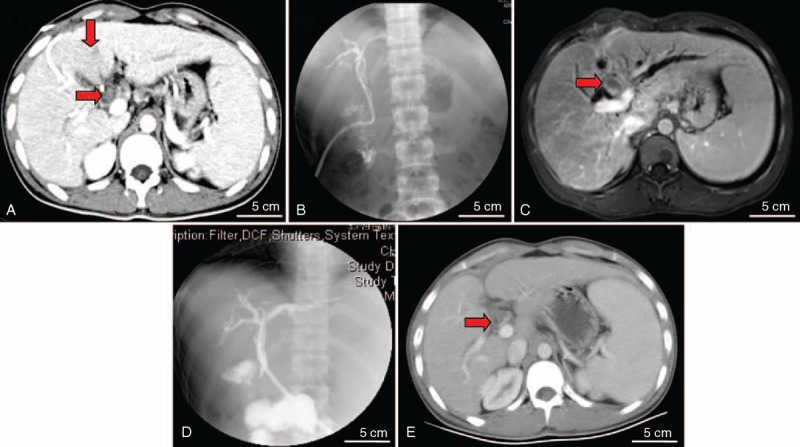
Image data of the patient who experienced BDTT re-recurrence after surgery. (A) HCC at segment 4 (vertical arrow) and tumor thrombus in common hepatic duct (horizontal arrow) were found with computed tomography. (B) Cholangiography showed that no definite filling defect was seen in the biliary system after hepatectomy and thrombectomy. (C) BDTT recurred (horizontal arrow) 7 months after first surgery (observed with functional magnetic resonance imaging). (D) Cholangiography showed that the recurrent BDTT was removed completely by reoperation. (E) Eight months after reoperation, computed tomography revealed that BDTT recurred (horizontal arrow) for the second time. BDTT = bile duct tumor thrombus, HCC = hepatocellular carcinoma.
